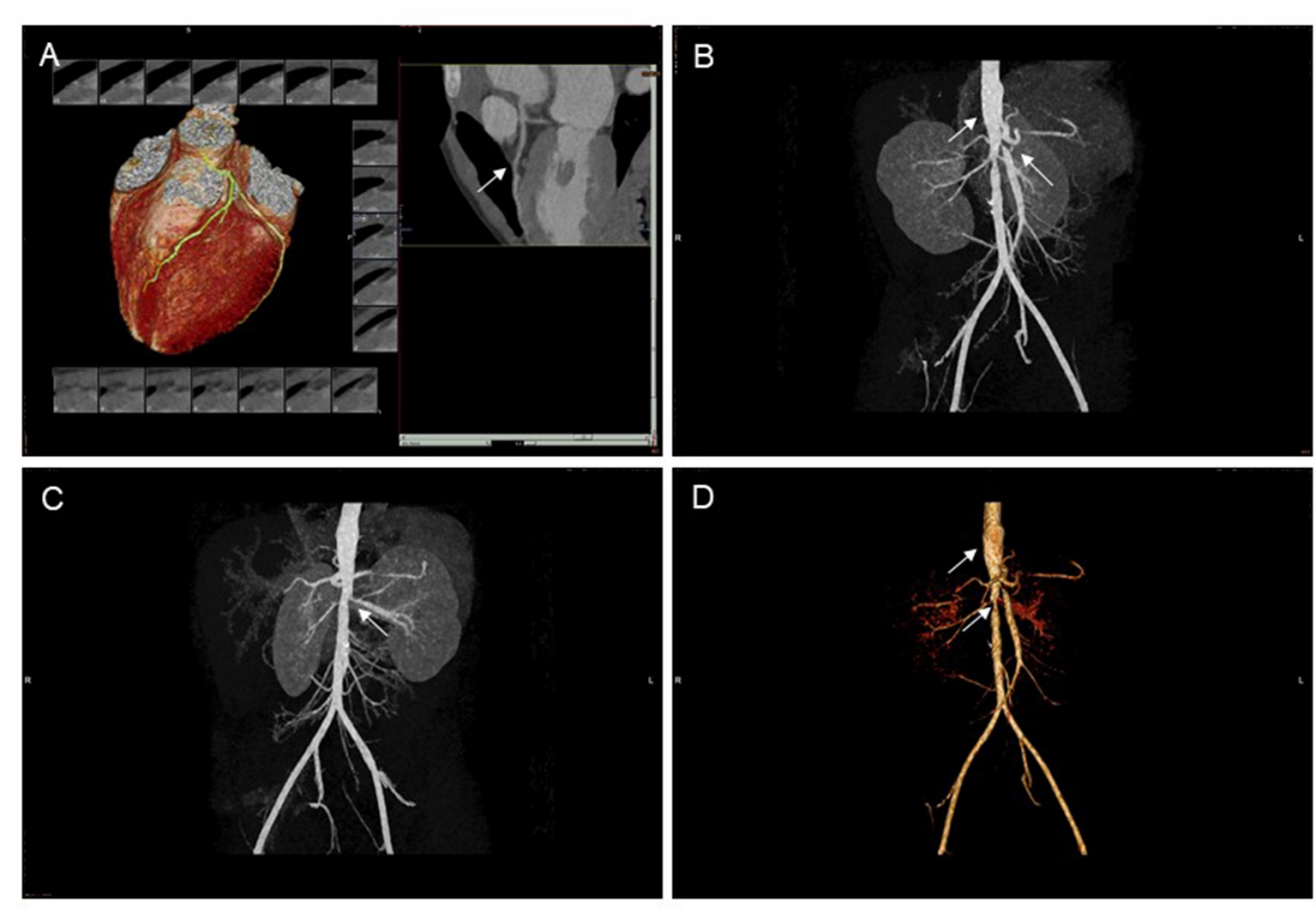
CT scan of coronary arteries and abdominal vessels. (A) Coronary artery stiffness, left coronary artery segmental stenosis. (B–D) The abdominal aortic wall is thickened and unevenly dilated, and the opening of the proximal bilateral renal artery, the celiac trunk and the superior mesenteric artery are narrowed. CT, computed tomography.
